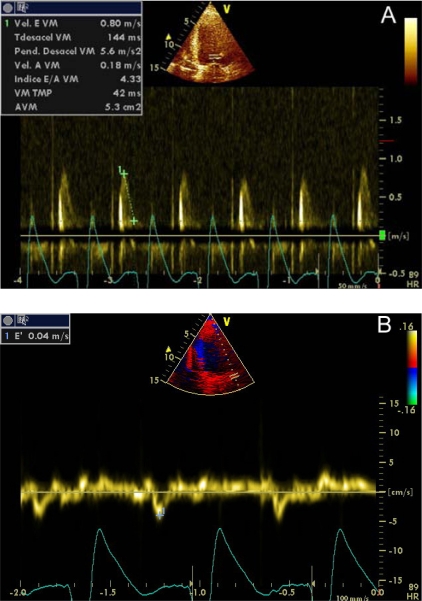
Third degree diastolic dysfunction (restrictive pattern). Transmitral pulsed Doppler (4) and Doppler Tissue Imaging (B). E/E´ ratio was 20.
